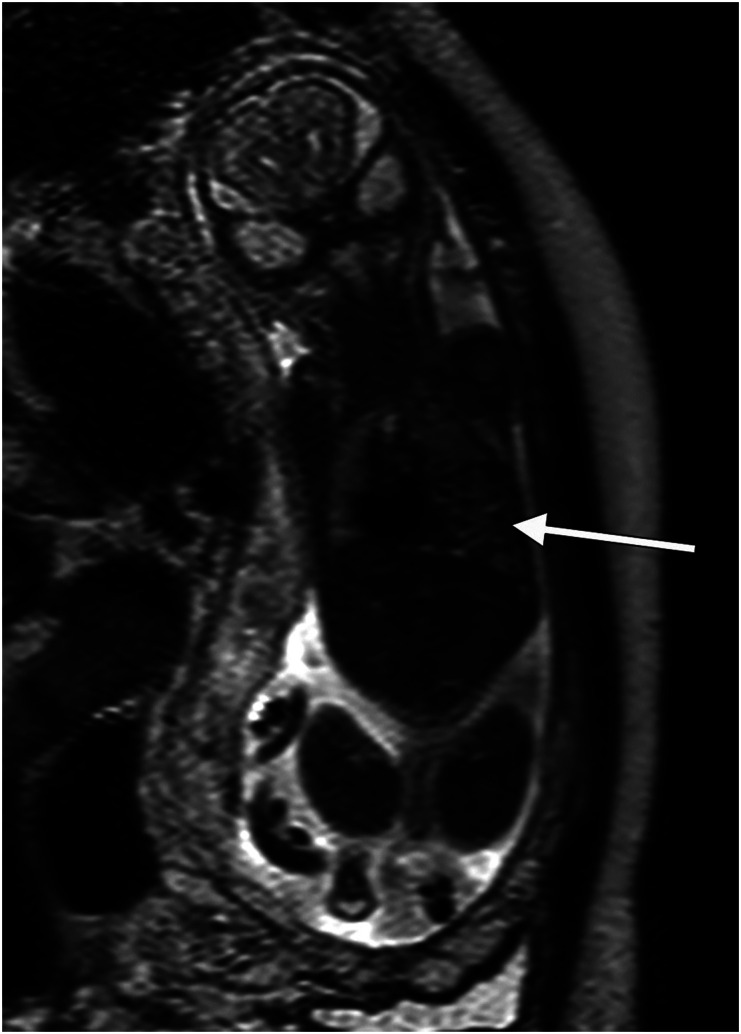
Case no 153. Ultrasound examination revealed right-sided heart but intact diaphragm. Coronal MR image shows left-sided diaphragmatic hernia containing intestines (arrow) but with normal position of the liver.
